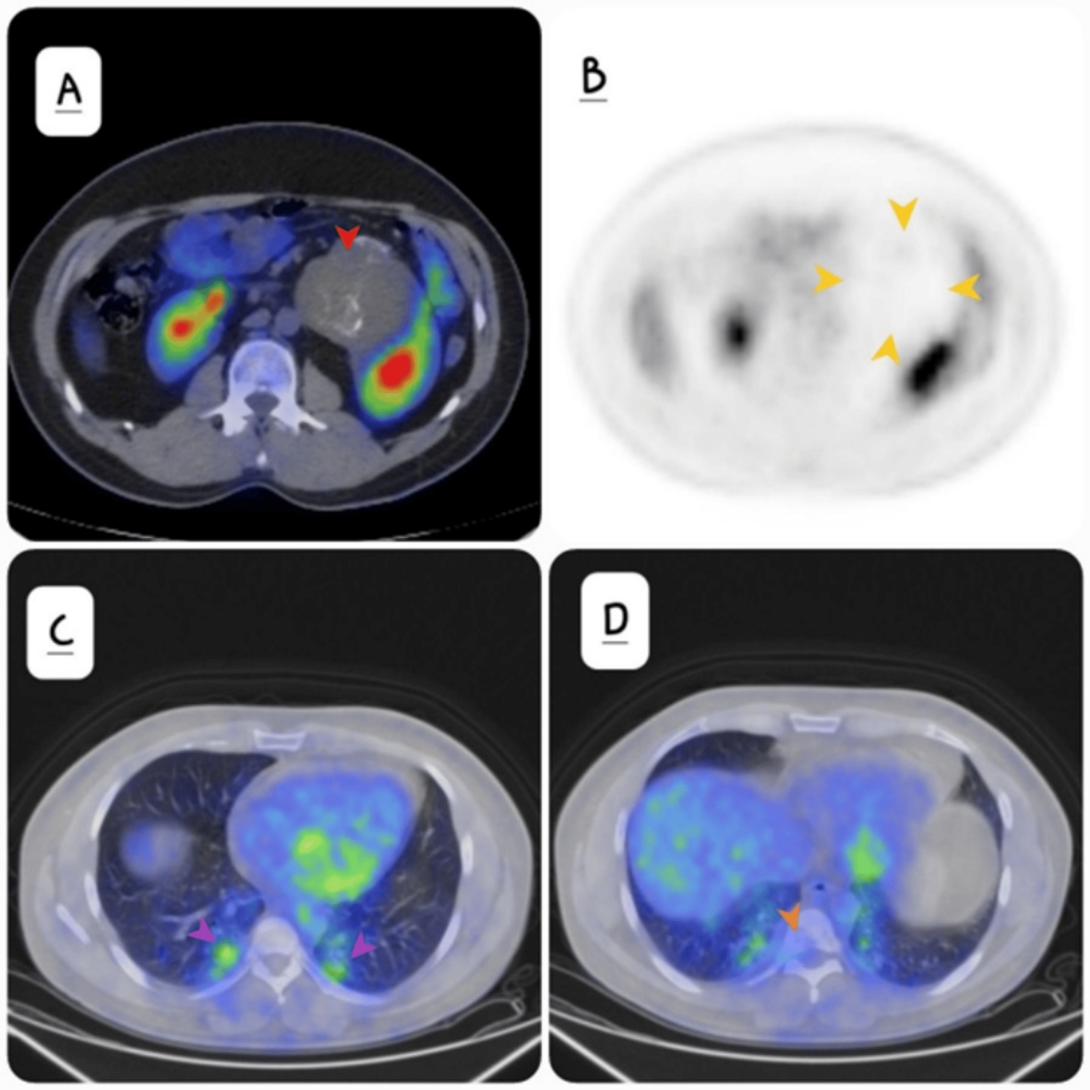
(A) Axial tomographic FDG PET-CT fusion image reveals the mass is hypo metabolic (red arrow). B) PET tomographic axial image confirms the mass is photopenic (yellow arrows). (C) Shows hypermetabolic lung metastasis (purple arrows) and (D) demonstrates hypermetabolic bone metastasis (orange arrow) FDG: Fluorodeoxyglucose
